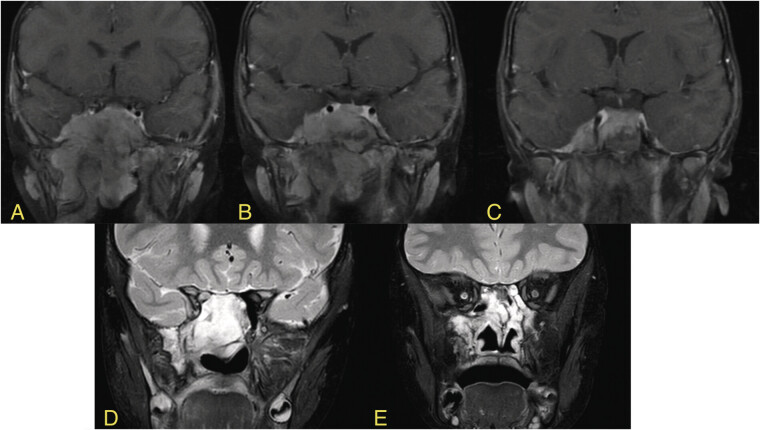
Serial coronal MRI images demonstrating treatment response over time. (
